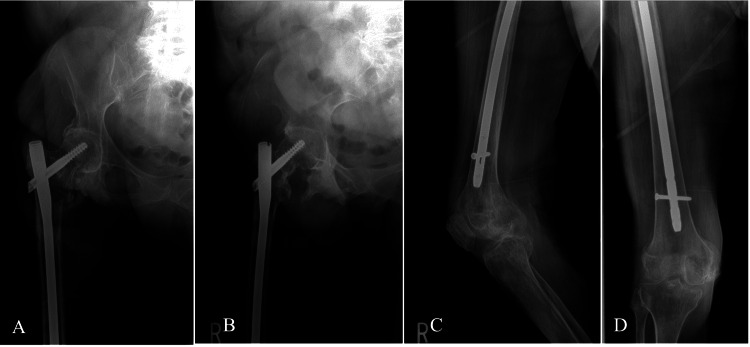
Six-week postoperative radiographs of the right femur A) Anteroposterior view of the right proximal femur. B) Lateral view of the right proximal femur. C) Anteroposterior view of the right distal femur. D) Lateral view of the right distal femur.
